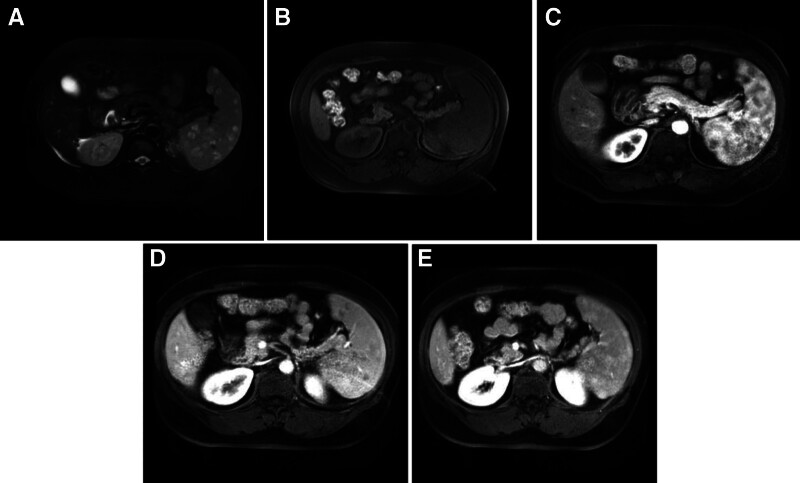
(A) T2WI showed lesions with a mixed high and low signal intensity. Within the high signal area, punctate low signals were observed, resembling a “freckle-like” appearance. (B) T1WI revealed iso-low hypointense signals with mixed intensity. (C) MRI enhanced the arterial phase with mild enhancement around the lesion. (D and E) MRI enhanced the portal phase and balance phase, with the further slow centripetal enhancement of the lesion. MRI = magnetic resonance imaging, T1WI = T1 weighted, T2WI = T2-weighted.
